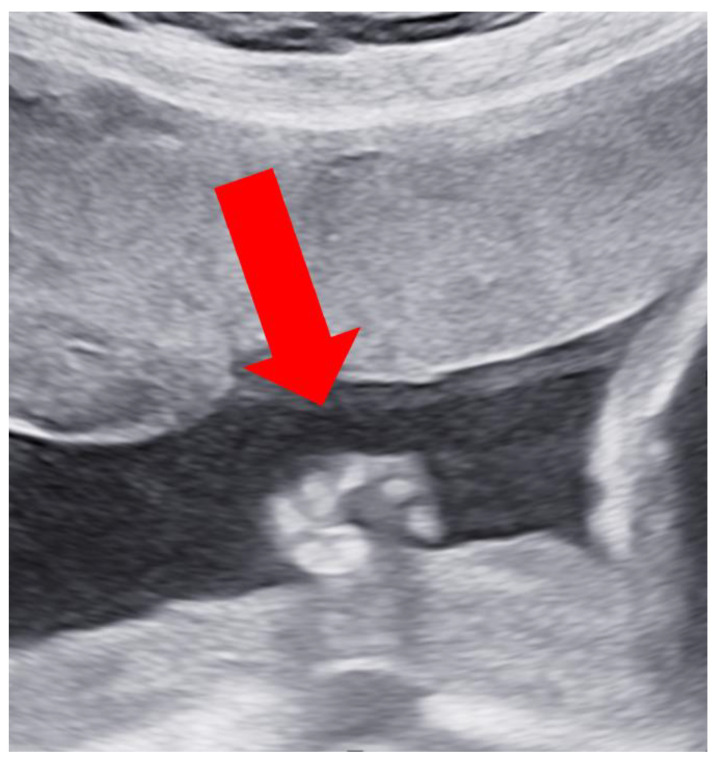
Clenched hand. Transabdominal ultrasound.
